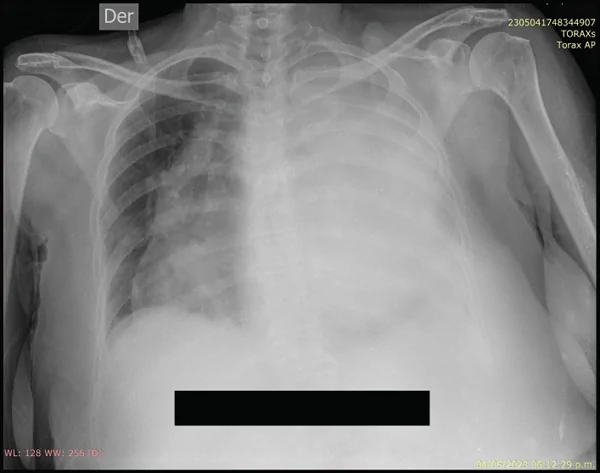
Chest radiograph demonstrating total opacification of the left hemithorax with mediastinal displacement.
